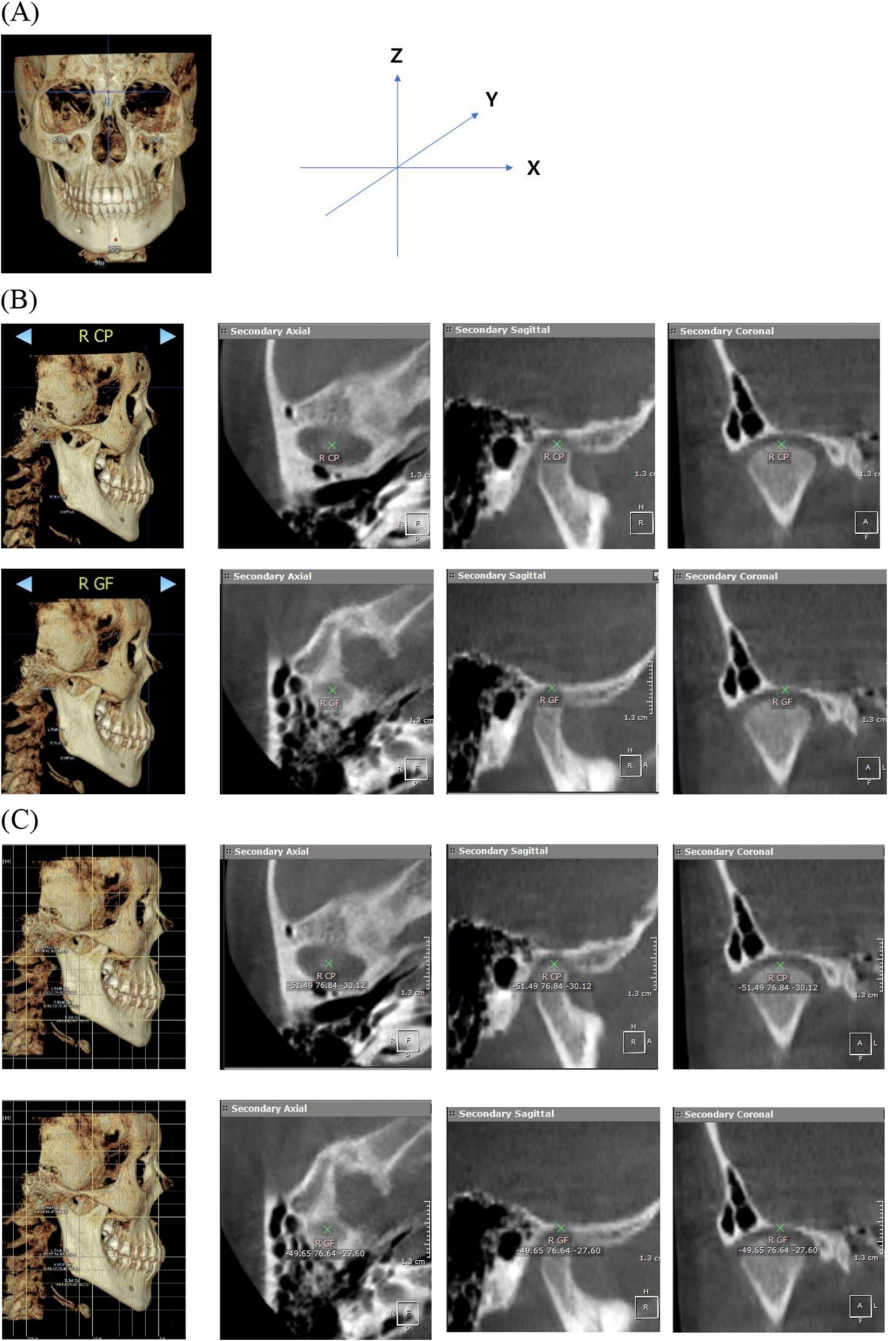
Data processing procedure. A Patients’ reconstructed 3D images were oriented based on the Frankfurt Horizontal (FH) plane method using Orbitale (Or) and Porion (Po). B On the axial, sagittal, and coronal sections of CT images, condylar point (CP) and glenoid fossa point (GF) of the affected and contralateral condyles were manually traced. C The *x*, *y*, and *z* coordinates of CP and GF of the affected and contralateral condyles were obtained
